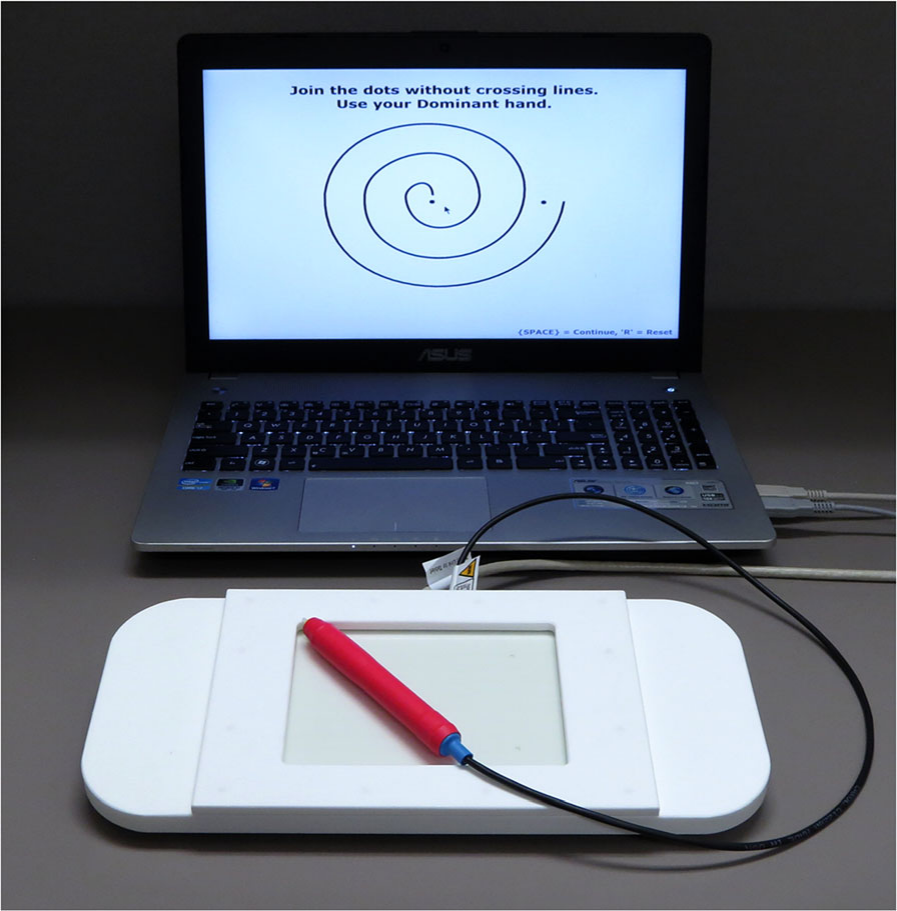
Testing computer and MRI-compatible tablet. The tablet was placed on the participant’s lap when seated for pre- and post-operative tests, and was supported by cushions on the participant’s abdomen for intra-operative testing during MRgFUS
